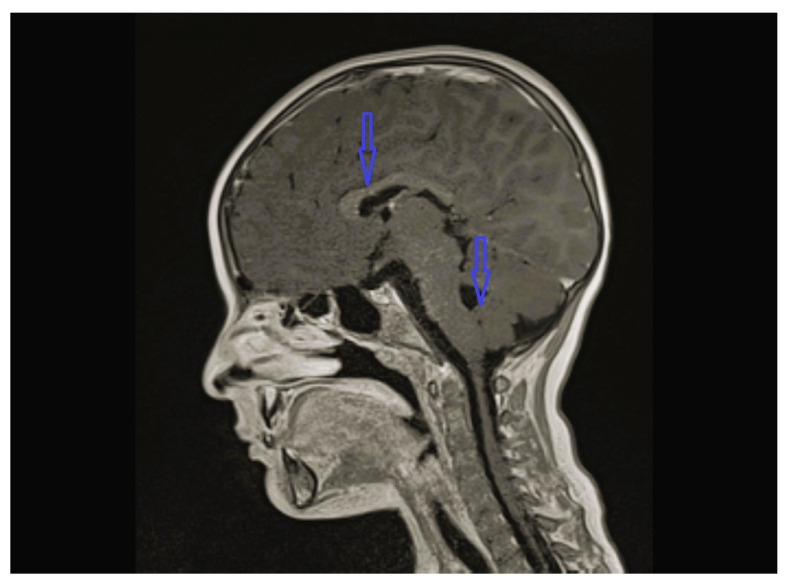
Brain MRI of case 1; the findings were consistent with hypoplasia of the vermis, cerebellum, and corpus callosum.
